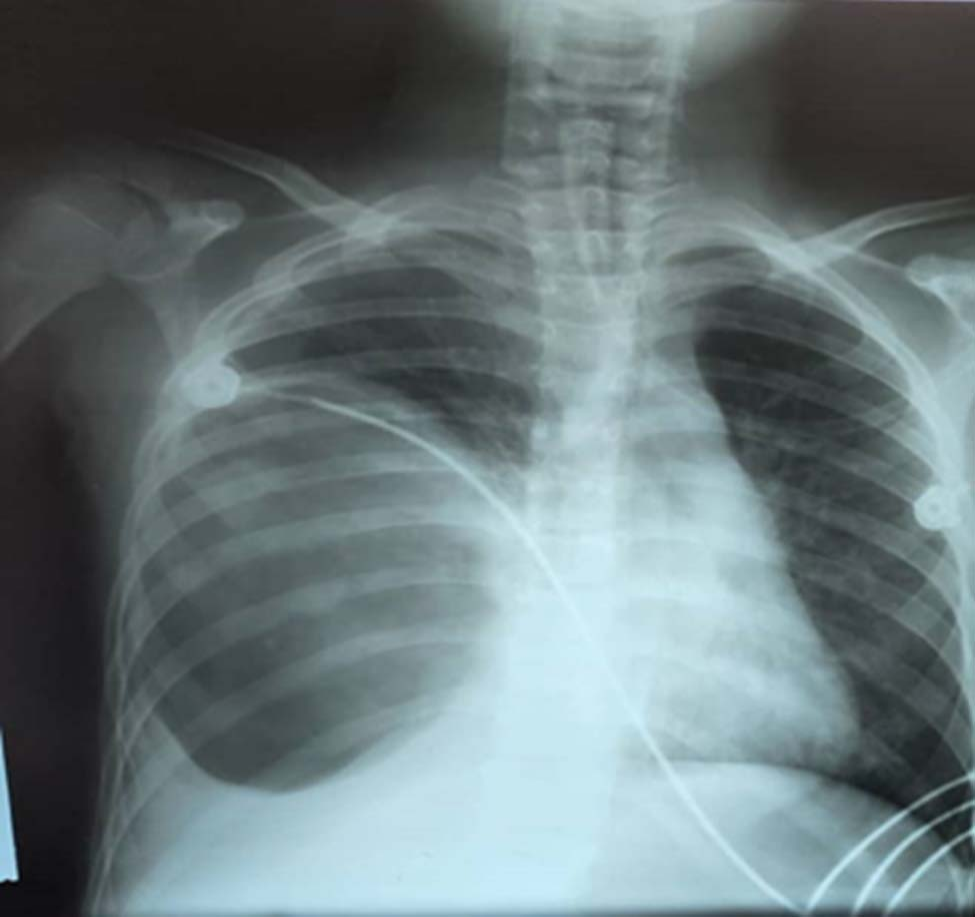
Depicts a chest X-ray image revealing a distinctive round, sharp-edged opacity situated in the lower region of the right lung, as highlighted by white arrows.
